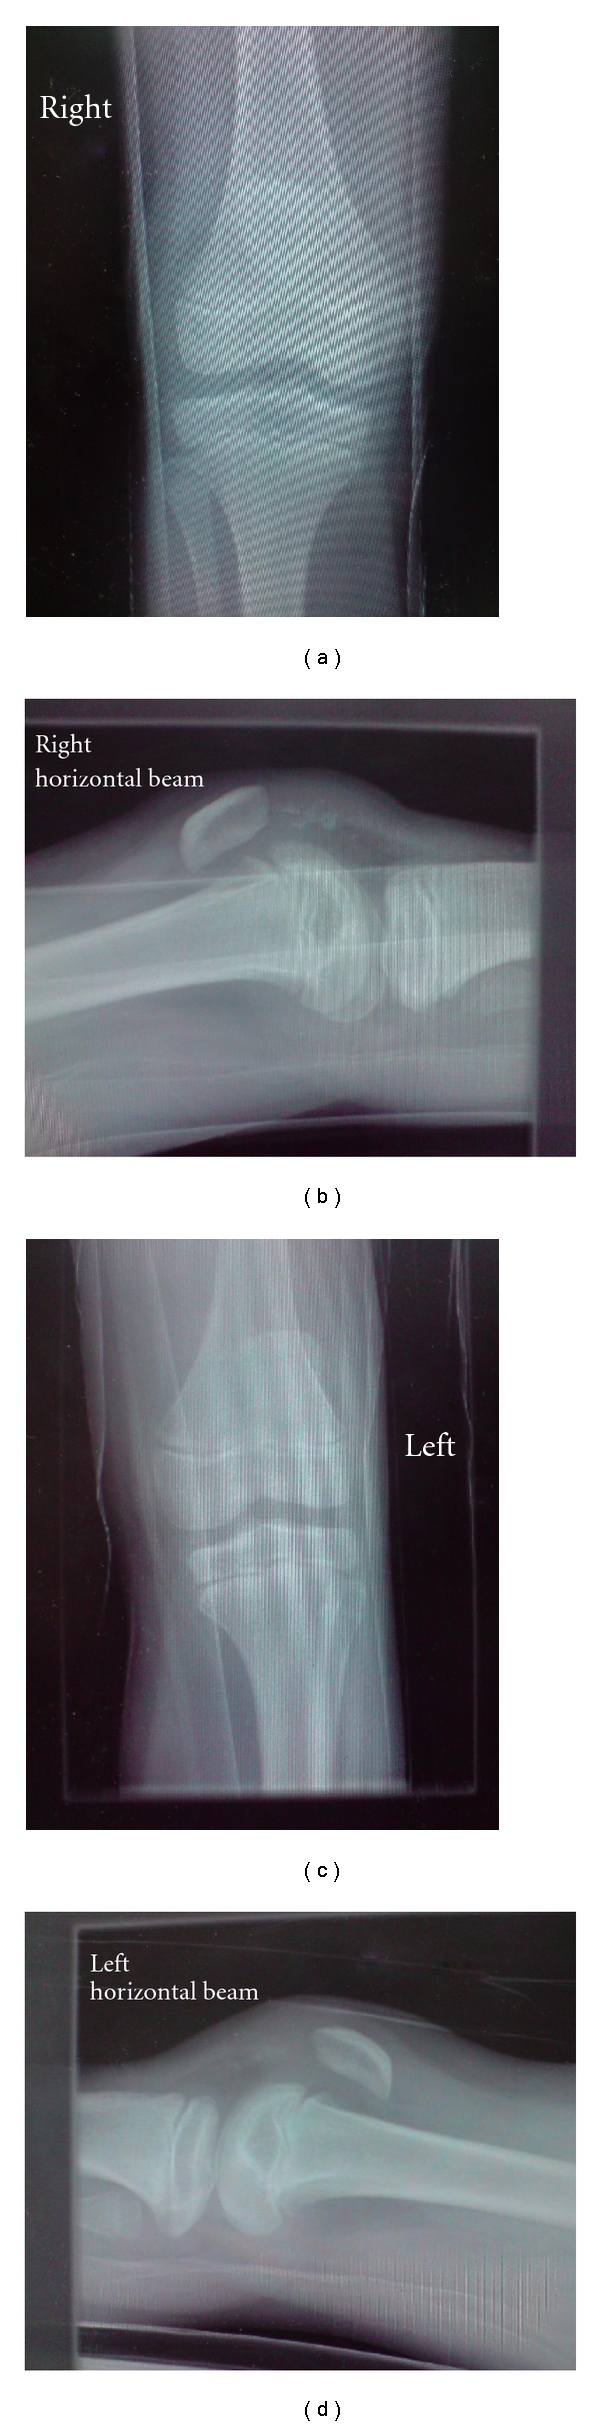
(a) AP radiograph of the right knee. (b) Lateral radiograph of the right knee. (c) AP radiograph of the left knee. (d) Lateral radiograph of the left knee.
